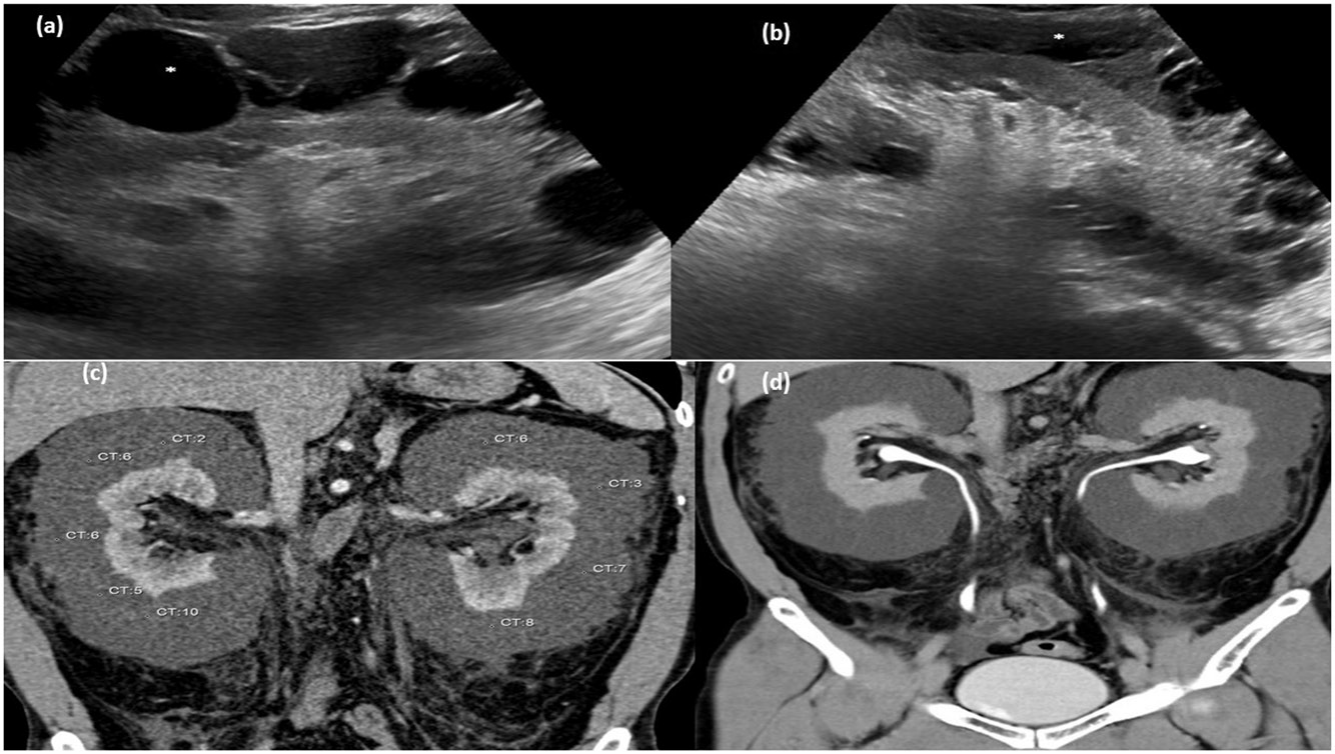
Gray-scale ultrasound image of right kidney (a) and left kidney (b) showing multiseptated anechoic collection (asterisk) in perinephric region. (c) CT scan arterial phase in coronal plane showing multiseptated nonenhancing collection with average HU density 0–10 HU. (d) MPR reformation of coronal plane of delayed phase of CT scan abdomen showing normal excretion of contrast.
